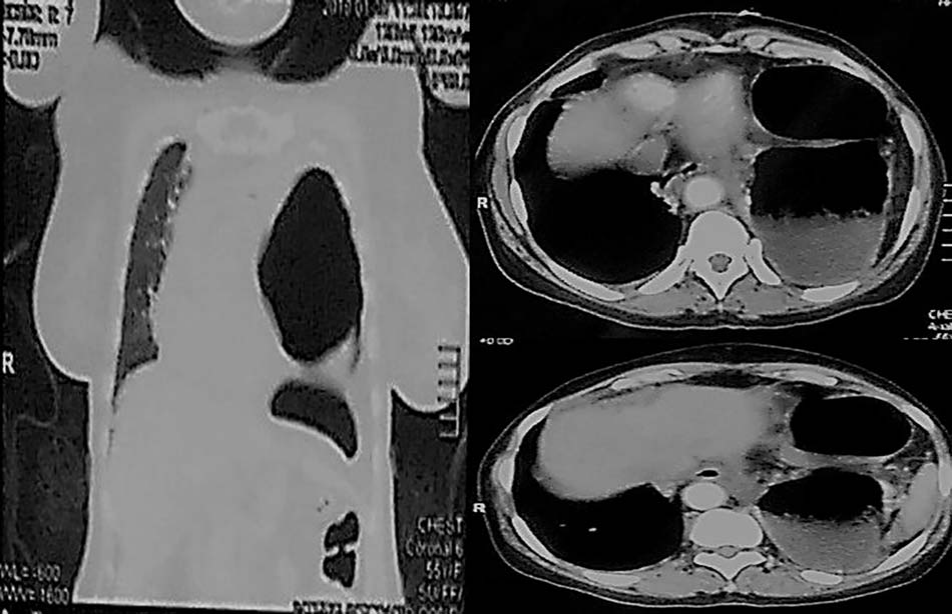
CT Thorax showing herniation of the stomach and spleen in the left chest cavity.
